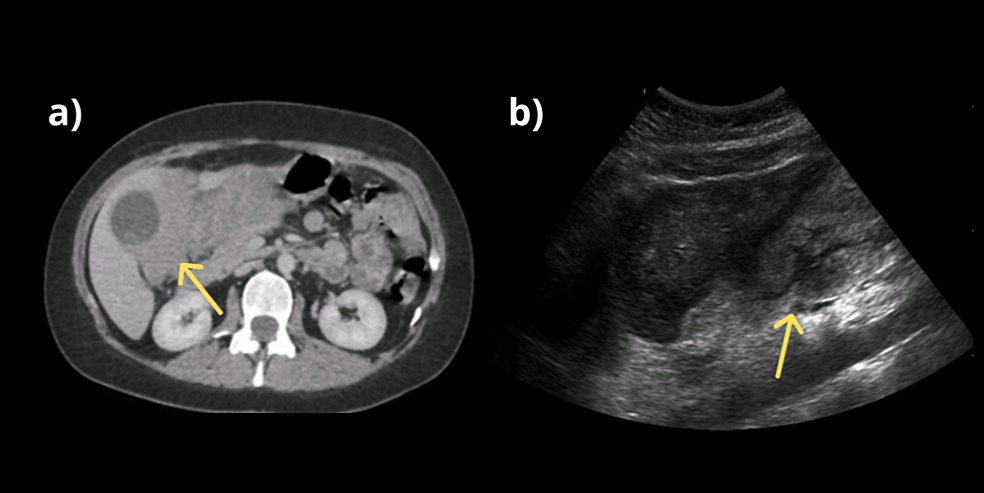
Initial scans on admission a) Initial CT scan showing a hypodense mass involving both liver lobes. b) Liver ultrasound showing a large, isoechoic mass
